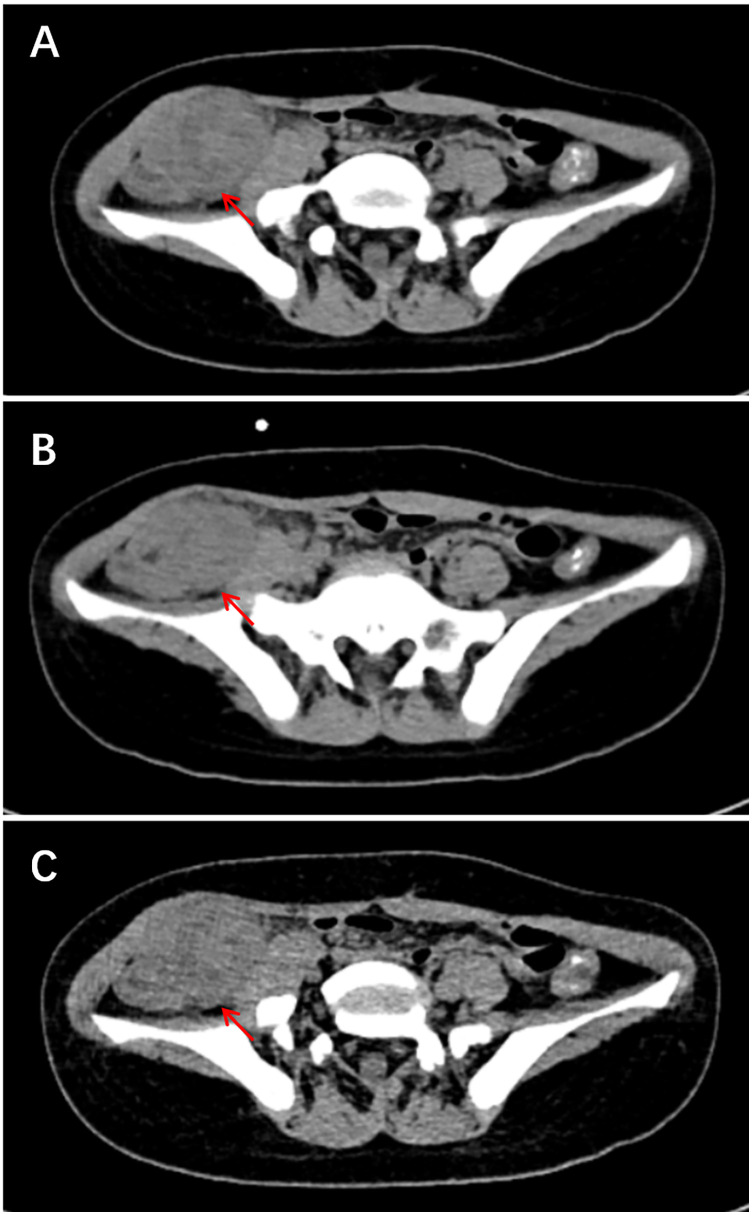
Ct images of right pelvic IMT. (A) CT image shows focal clustering of bowel loops in the right iliac fossa with an associated heterogeneous mass. (B–C) Coronal and sagittal reconstructions reveal the lesion measures approximately 4.1 cm × 3.9 cm and is accompanied by blurring of the surrounding fat planes.
